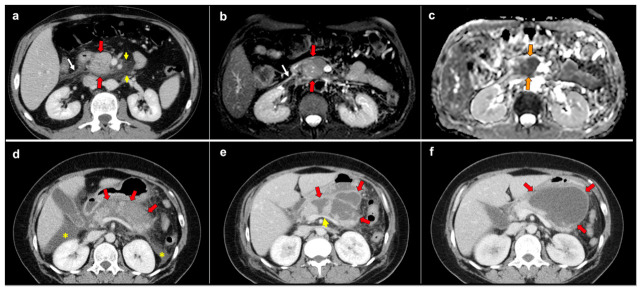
Two representative cases of acute pancreatitis. (a–c) Focal oedematous pancreatitis in a male patient with acute epigastric pain radiating to the back. (a) Axial portal-phase CT shows enlargement of the pancreatic head with slightly heterogeneous enhancement (red arrows), ill-defined stranding of the adjacent fat (yellow arrowheads) and a small amount of fluid in the right anterior pararenal space (white arrow). (b) Axial fat-saturated T2WI shows mild hyperintensity of the pancreatic head reflecting inflammatory changes (red arrows) and the right pararenal fluid (white arrow). (c) ADC map shows focal restricted diffusion with low ADC values (orange arrows). (d–f) Necrotising pancreatitis in a female patient with acute abdominal pain. (d) Axial portal-phase CT shows diffuse acute pancreatitis with ill-defined areas of hypoenhancing parenchyma due to oedema and glandular necrosis (red arrows), associated with stranding of the adjacent fat and inflammatory exudates extending towards the anterior pararenal spaces (yellow asterisks). (e) Follow-up CT obtained 10 days later shows partial replacement of the pancreatic parenchyma and peripancreatic fat by necrotic collections (red arrows), with stenosis of the splenic vein (yellow arrowhead). (f) Follow-up CT after 4 weeks shows confluence of the previous collections forming a large pseudocyst abutting the pancreas and stomach (red arrows), which required subsequent drainage by cystogastrostomy.
